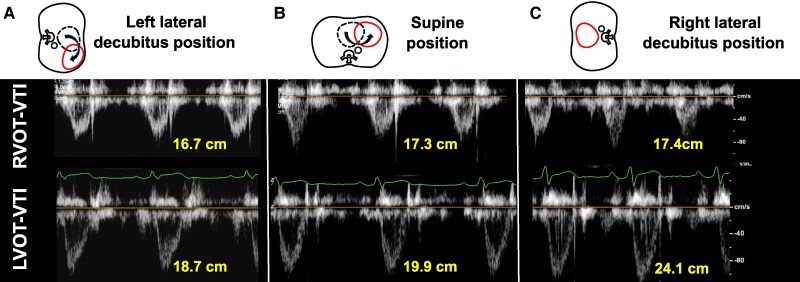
Doppler echocardiogram from RV and LV outflow tract of a 29-year-old man in various body positions. Compared with the right lateral decubitus position (*C*, right panel), the RV and LV outflow tract velocity-time integrals were significantly decreased in the supine position (*B*, middle panel) and further decreased in the left lateral decubitus position (*A*, left panel). LVOT, left ventricular outflow tract; RVOT, right ventricular outflow tract; VTI, velocity-time integral.
